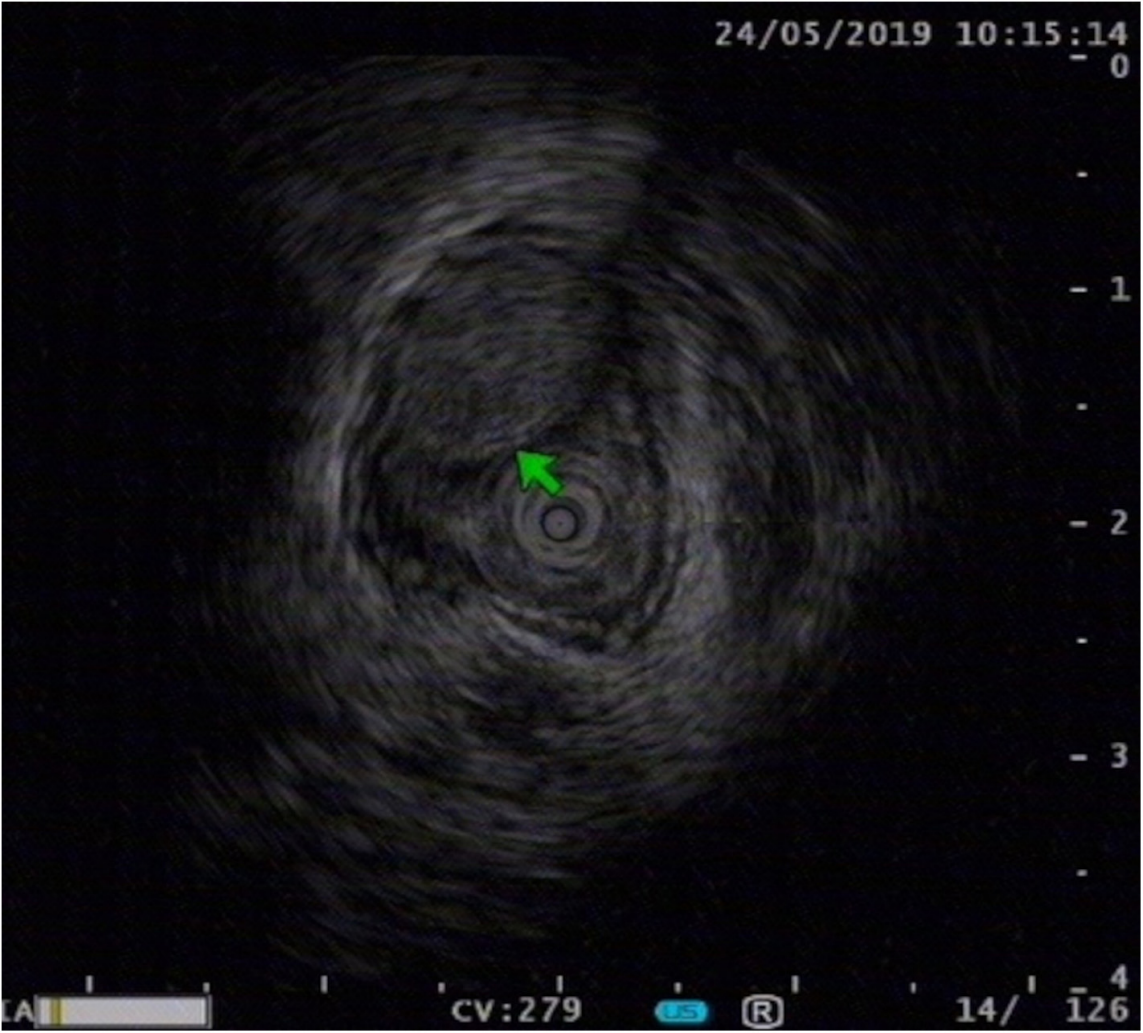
Endoscopic ultrasound revealing a hypoechoic lesion in the submucosal layer with a characteristic sieve-like appearance (arrow). The muscularis propria is intact.
